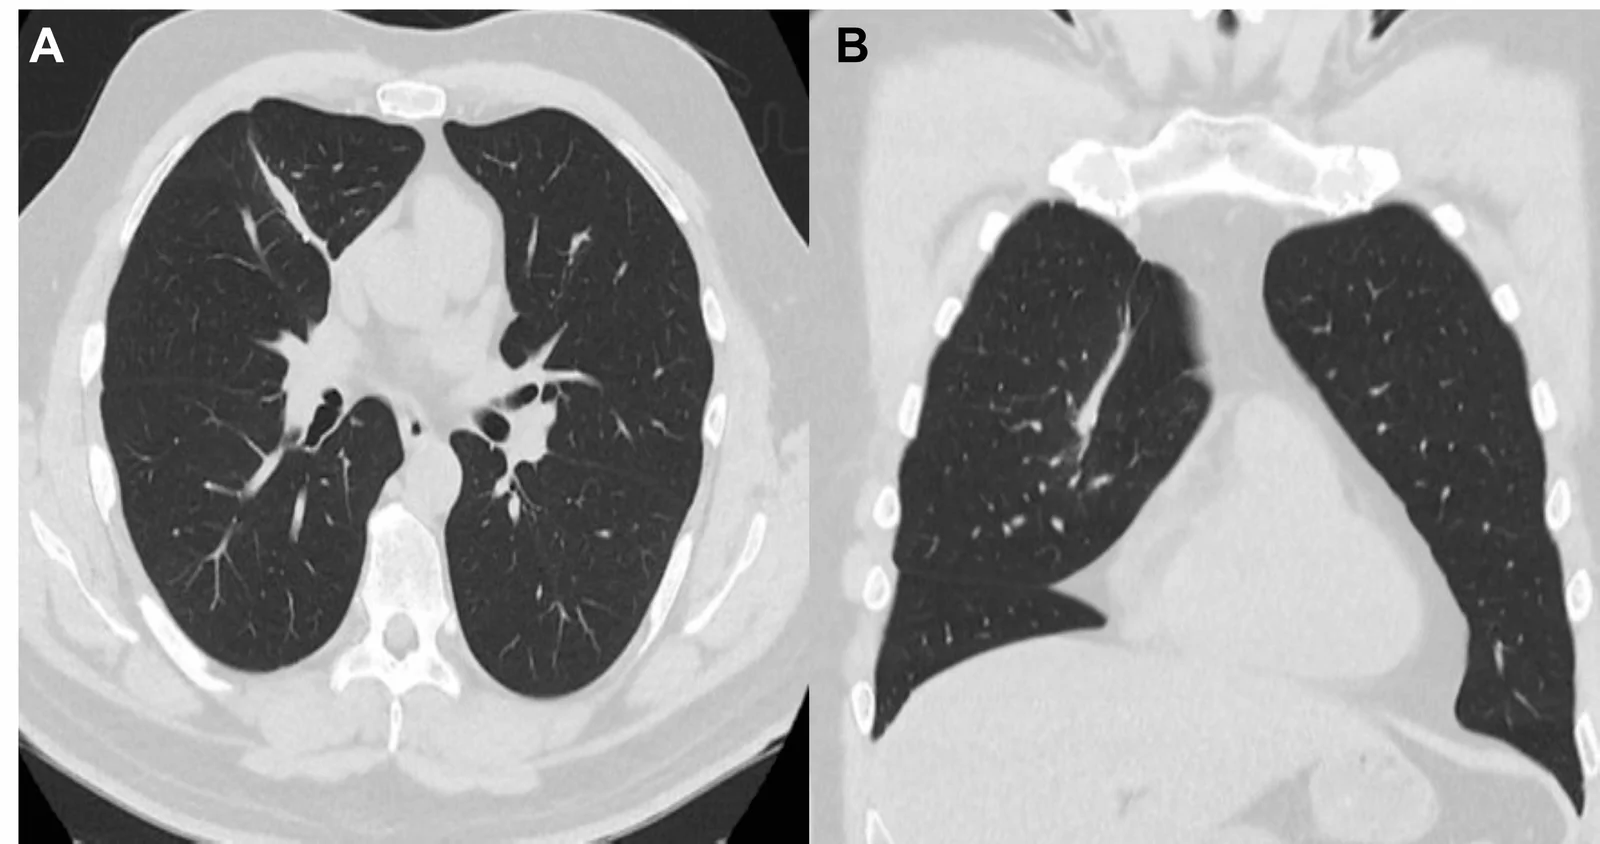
Postoperative CT of the chest following wedge resection. Axial (A) and coronal (B) high-resolution CT images demonstrating postoperative changes in the right upper lobe with linear scarring and no residual cystic lesion or suspicious pulmonary nodule.
